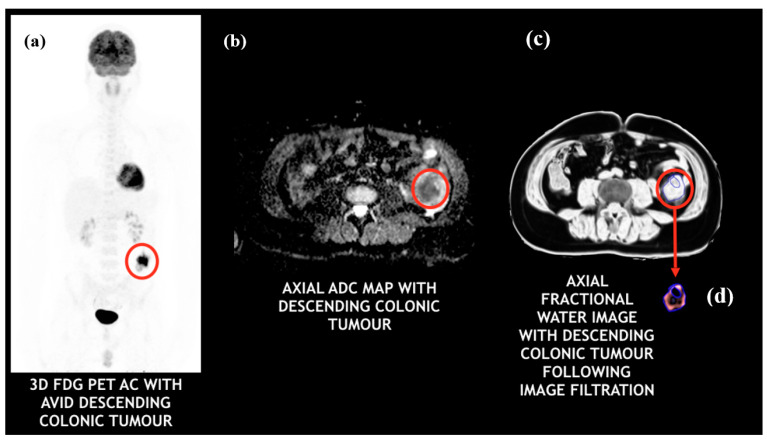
PET/MRI images of a colorectal cancer patient with a descending colonic tumor: (a) FDG-PET (tumor appears to be intensely avid); (b) ADC map (tumor appears to be highly cellular); (c) fractional water image (tumor appears to have very high water content); (d) fractional water texture map following coarse image filtration (bright/intense objects representing pockets of high water distribution within the tumor).
